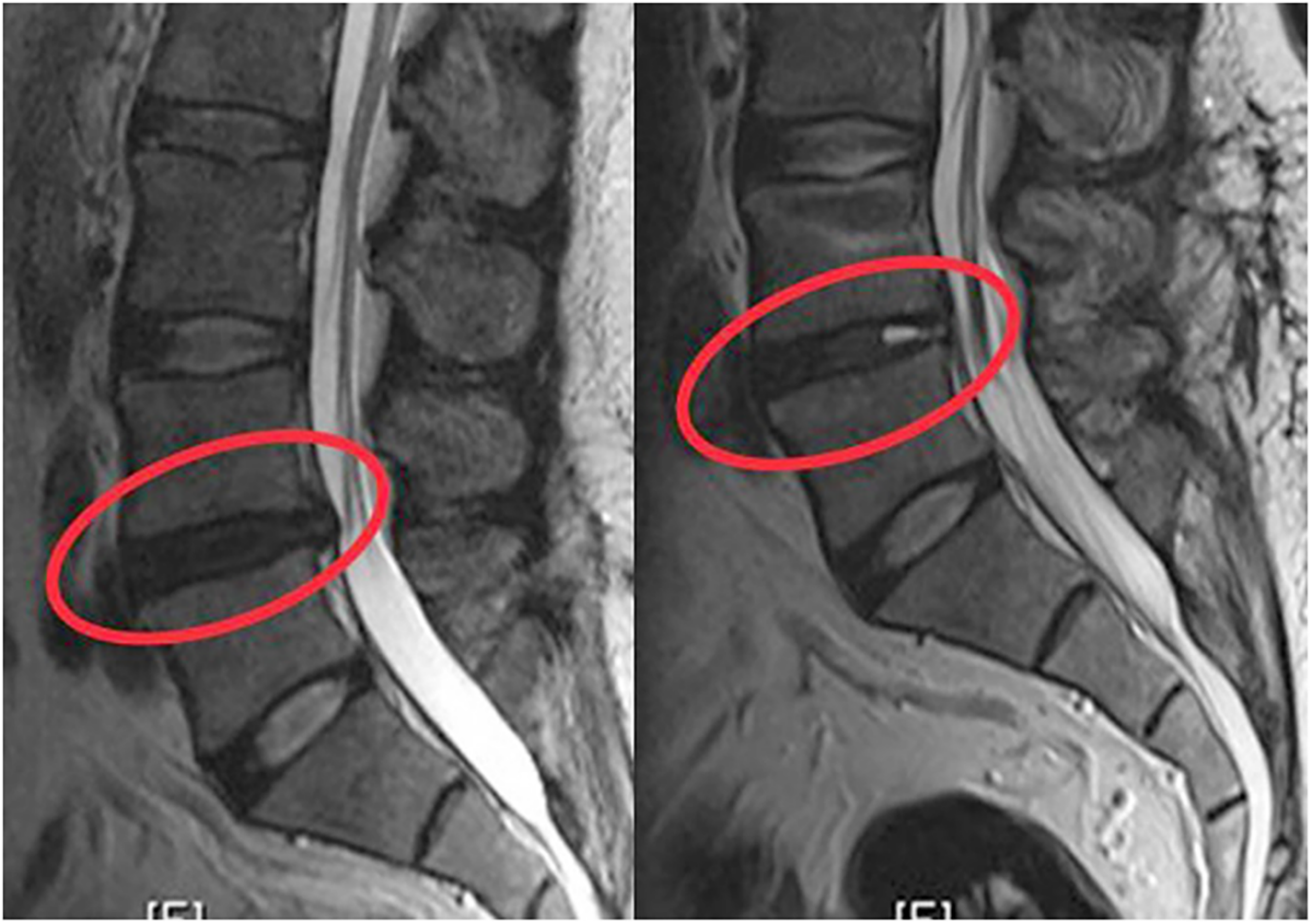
The patient with painful degenerative disc disease was grade 4 according to Pfirrmann classification due to decreased signal intensity in the preoperative T2-weighted MRI. After dynamic stabilization with Safinaz screw and titanium rod, the patient was evaluated as grade 3 due to the increase in intensity in the T2-weighted MRI taken at the 12th month.
